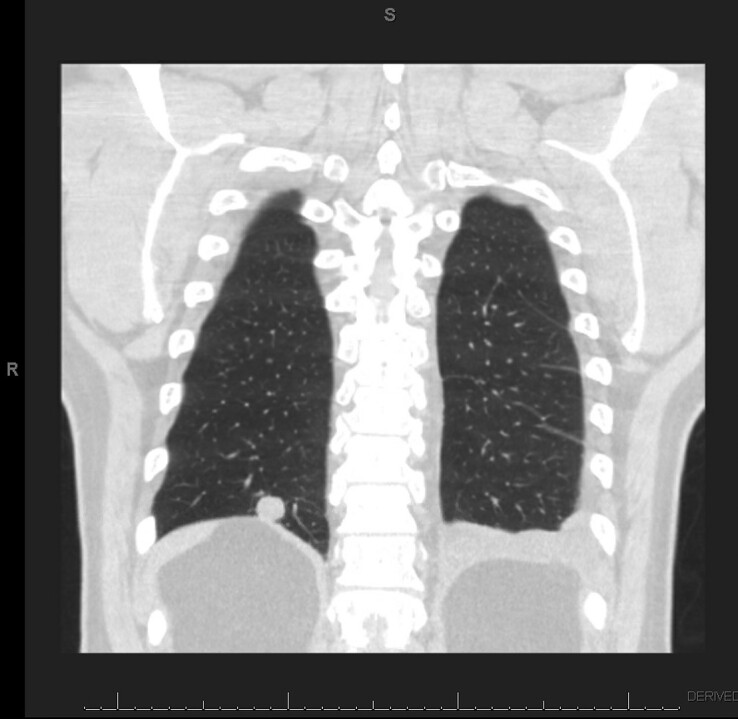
Chest CT obtained in April 2019. Coronal cross-section with right lower lobe nodule and left effusion. Abbreviation: CT, computed tomography.
